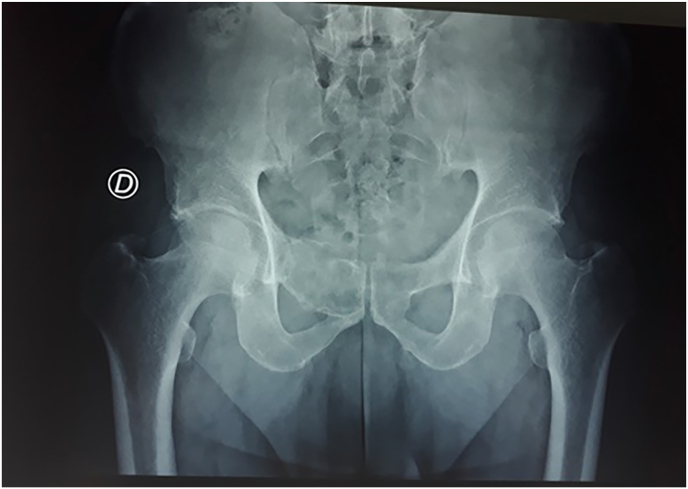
Anterior-posterior X-ray of the pelvis showing an osteolytic lesion of right superior pubic rami.
